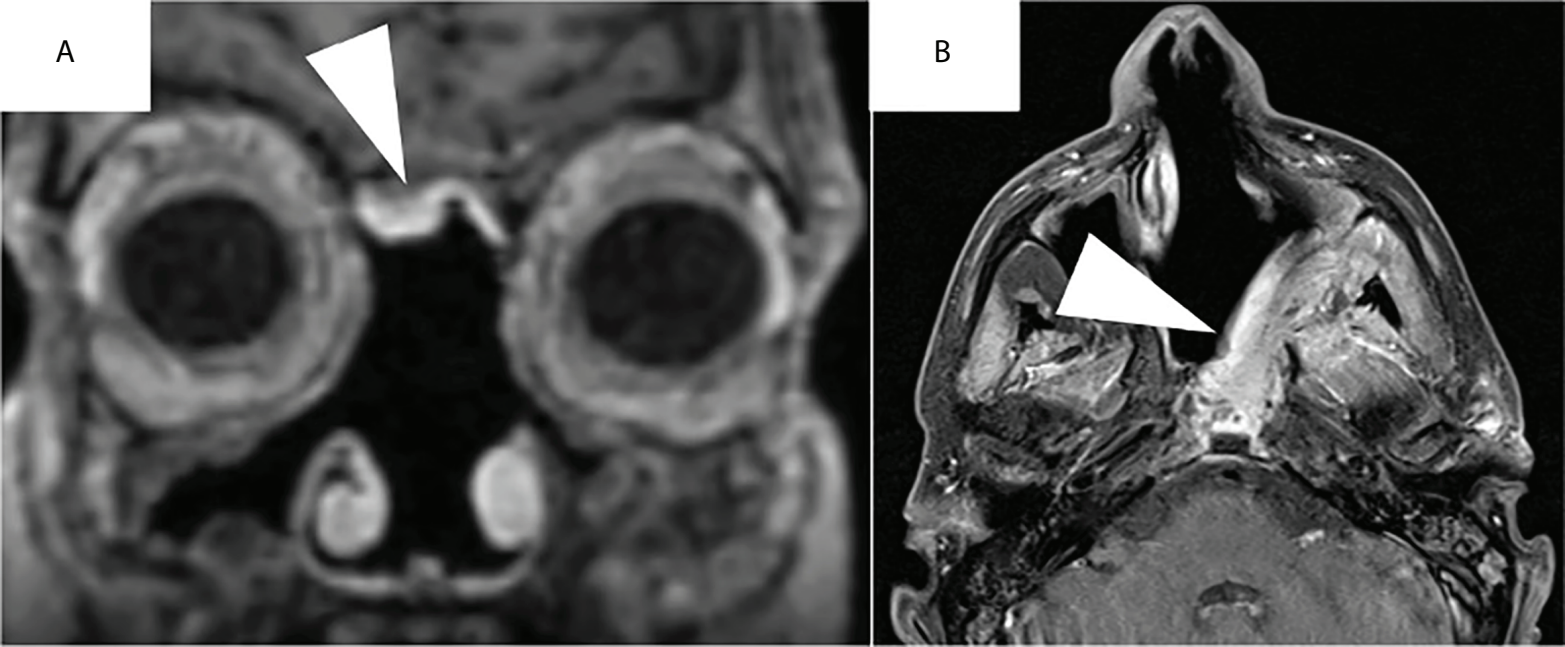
Post-operative aspect of a nasoseptal flap (A) and of a left superficial temporoparietal fascia flap (B) on contrast-enhanced T1-weighted MRI sequences. Note the hyperintense aspect of the flaps after contrast enhancement (white arrowheads).
